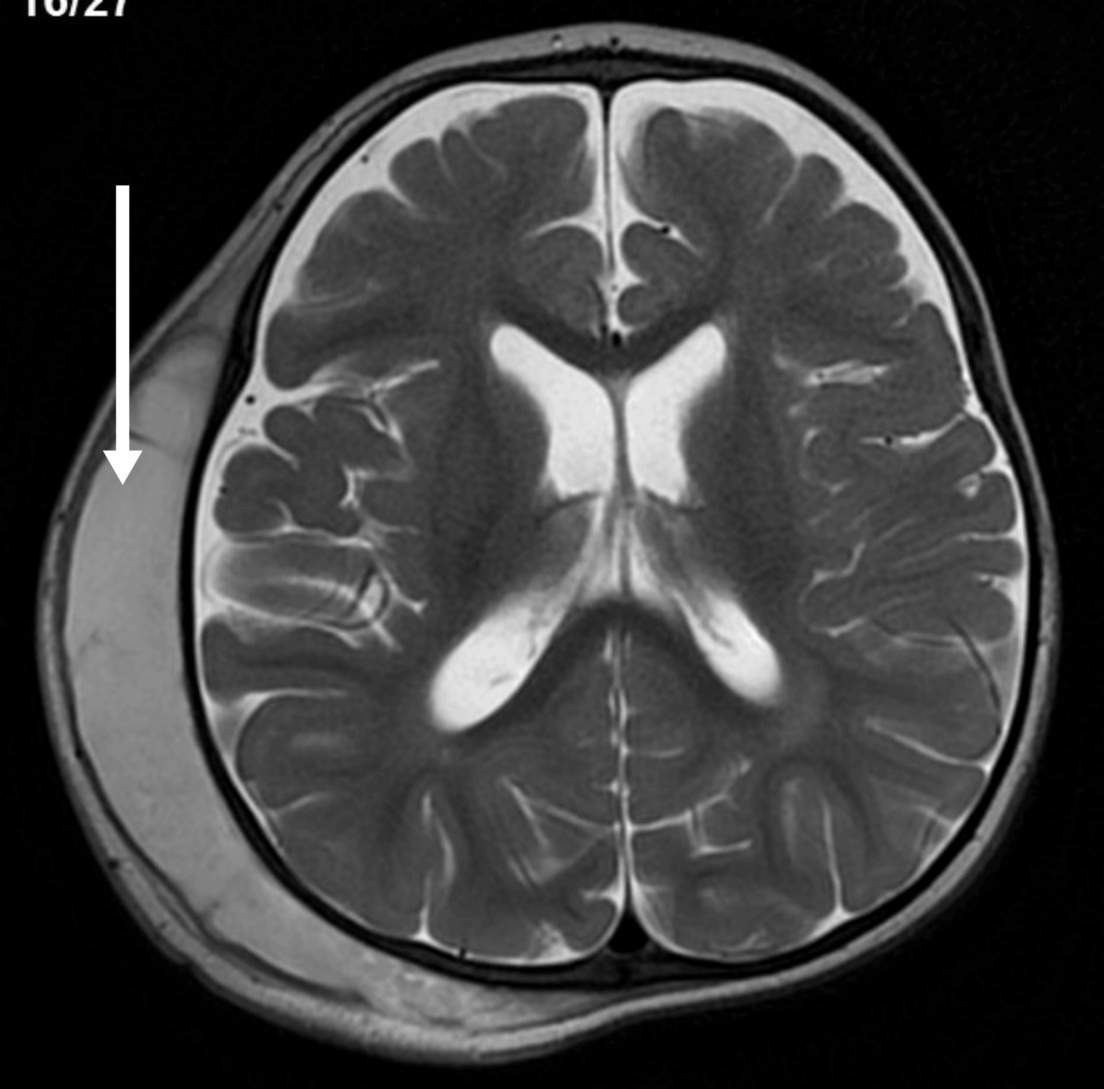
Post-contrast MRI of the brain: axial T2 WI MRI brain showed subgaleal fluid collections with bright signals and few septations
